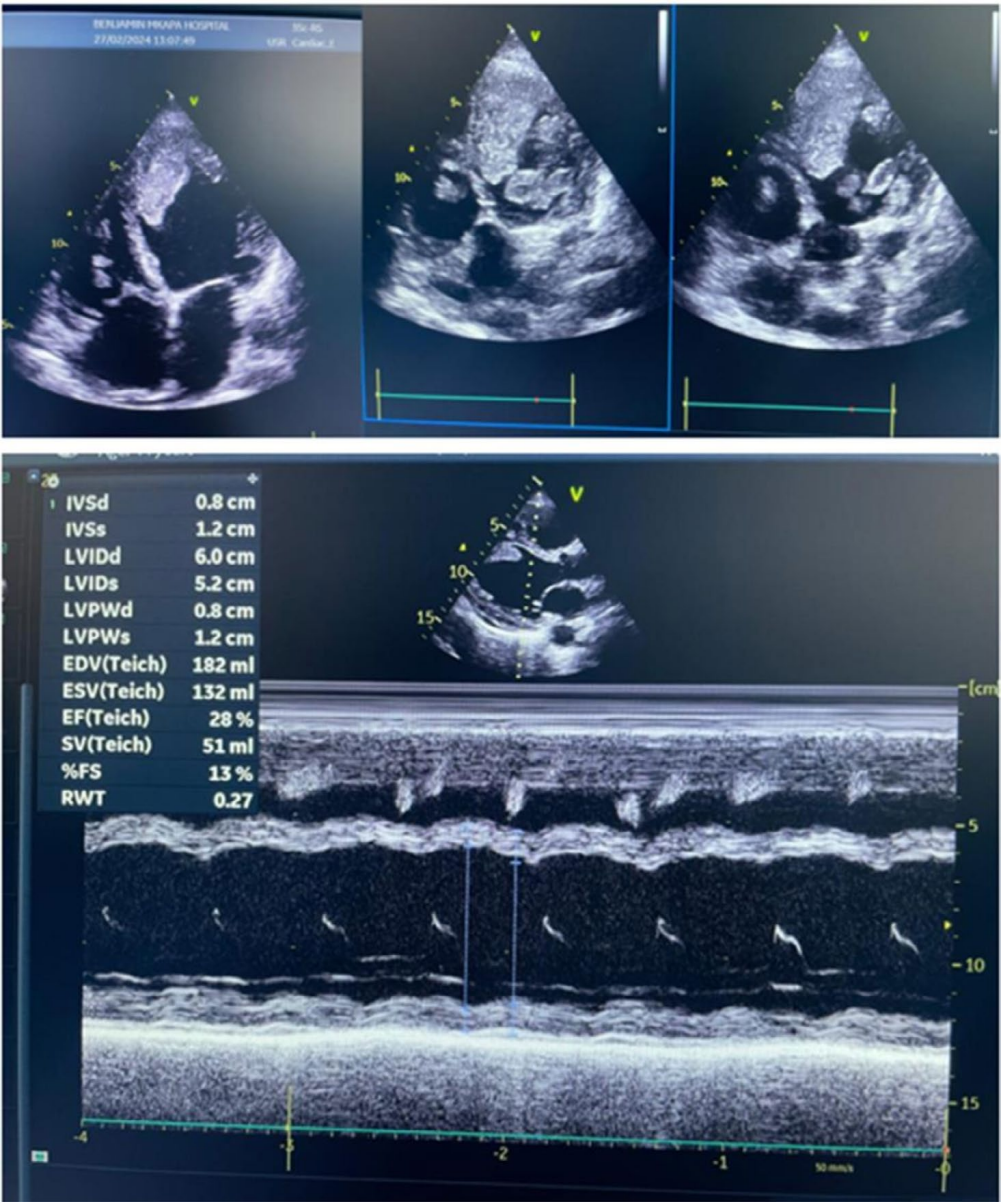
2D 4‐chambers view showing multiple intracardiac thrombi on both ventricles. 2D‐parasternal long axis view M‐mode showing EF of 28% (during admission).
